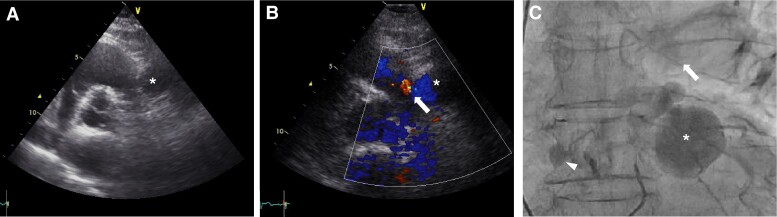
Transthoracic echocardiography showing a round cystic lesion (*) located in front of the right ventricular outflow tract (*A*) and efferent flow into the pulmonary artery (arrow) on colour Doppler flow imaging (*B*). Coronary angiography of the left coronary artery shows both a small aneurysm of the right coronary artery (arrowhead) and the coronary pulmonary artery fistula connecting the proximal branch of the left anterior descending artery to the main pulmonary artery (arrow) through the large aneurysm (*) (*C*).
